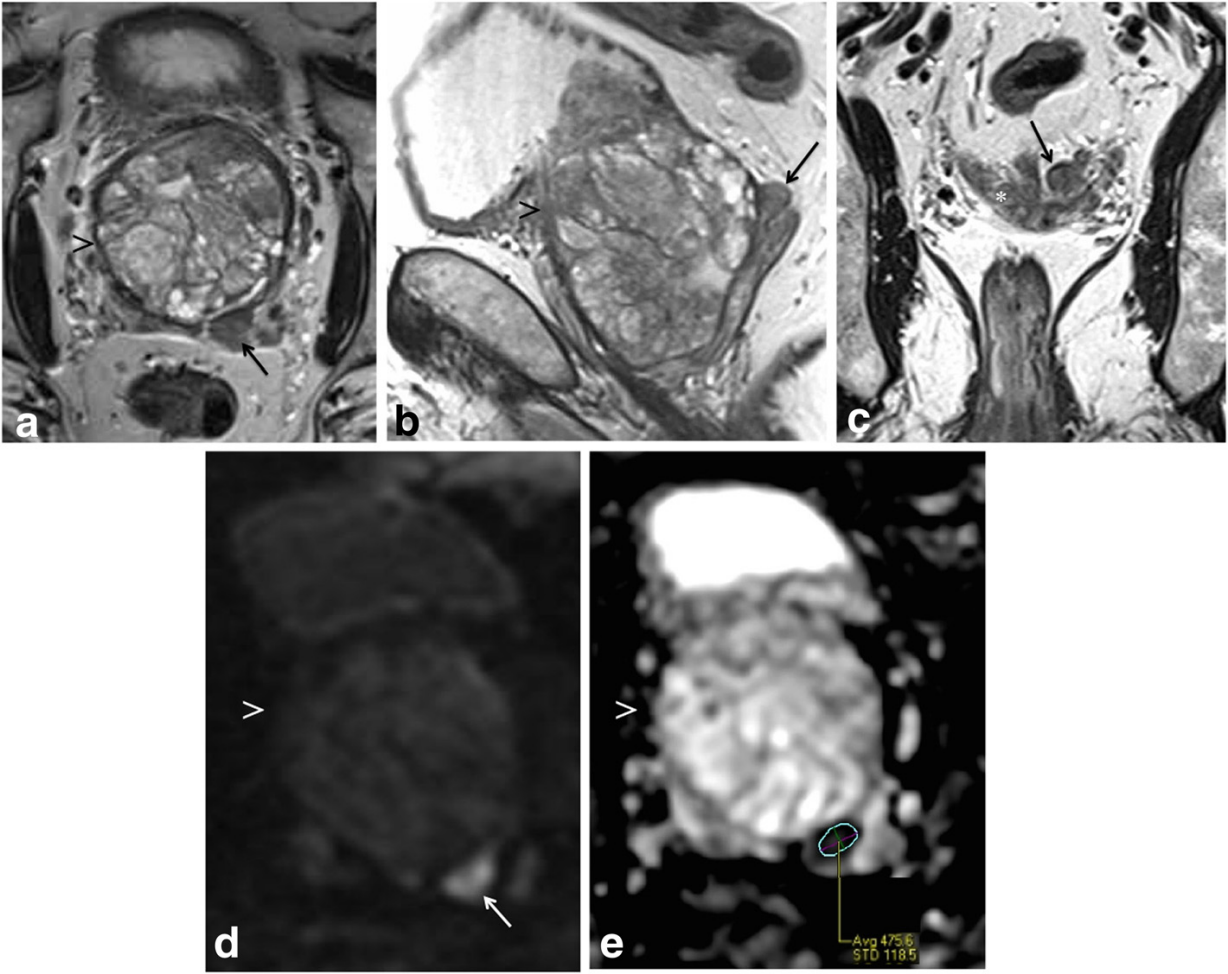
Multi-parametric magnetic resonance (MR) imaging of the prostate gland demonstrates normal prostate gland however an abnormal lesion in the left seminal vesicle features classical imaging features of prostate carcinoma. a High-resolution axial T2-weighted (T2W) image demonstrates an abnormal 1.5 × 1.2 × 1.0 cm lesion with relative hypointensity (*arrow*) subjacent to an enlarged prostate gland (*arrowhead*). The capsule is maintained without trans-capsular extension. b–c High-resolution multi-planar T2W images in the sagittal and coronal planes show, to advantage, this lesion within the left seminal vesicle (*arrow*), external to the capsule of the prostate gland (*arrowhead*). No T2W signal intensity abnormality is in the adjacent left prostate gland base peripheral or transitional zone. Moreover, there is no exophytic hyperplastic nodule or contiguous extension of prostatic glandular tissue related to the abnormality in the left seminal vesicle. Normal right seminal vesicle denoted for comparison (*asterisk*). d High *b*-value diffusion weighted imaging (DWI) shows that this lesion demonstrates significantly diminished diffusivity (*arrow*). e Apparent diffusion coefficient (ADC) map confirms significantly diminished diffusivity in this lesion (*arrow*) with low ADC mean value of 0.5 × 10^−3^ mm^2^/s (*region of interest*). No diminished diffusivity was seen in the prostate gland (*arrowhead*)
